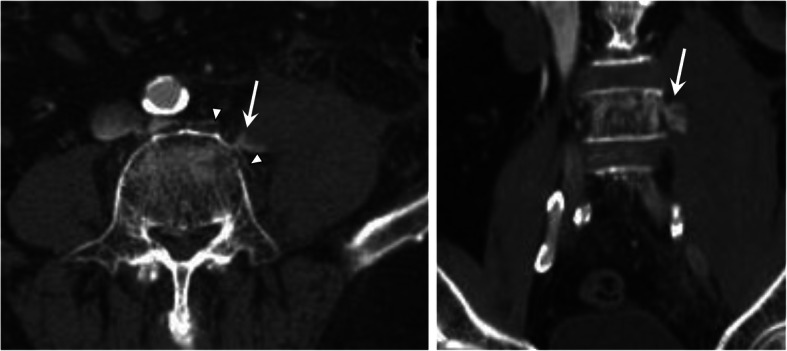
Computed tomography angiography on the day of admission. The leakage of the contrast medium (arrow) from the vertebral segmental artery (arrowhead) that runs around the fractured L4 vertebra is revealed
